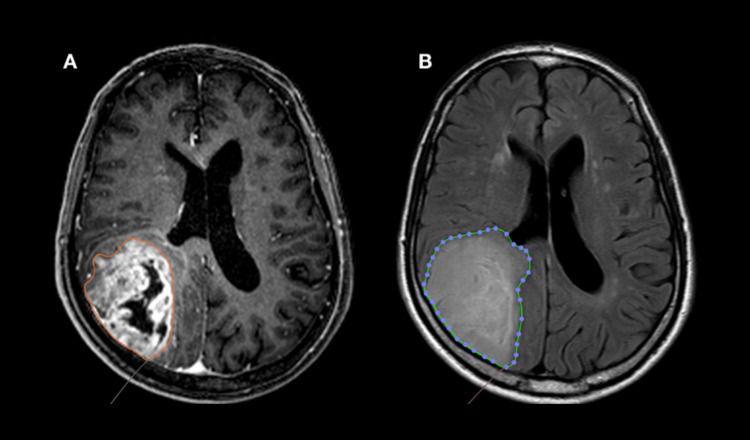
Glioblastoma multiforme MRI volumetric analysis a- MRI in T1-weighted sequence with gadolinium showing the calculation of lesion volume for enhancing zone. b - MRI in FLAIR-weighted sequence showing the calculation of lesion volume for tumor edema zone. Volumetric calculations were estimated with a manual tracer of the Horos software, by measuring the area of interest with a slice-by-slice semi-automatic method
